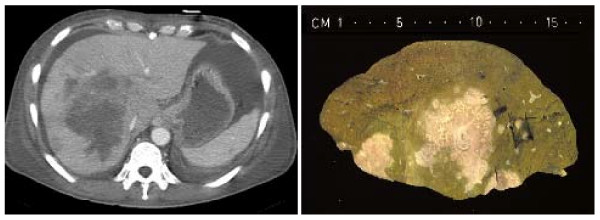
A. CT scan of the abdomen showing a large mass central in the liver, with extension to the right liver lobe. B. Postmortem aspect of the liver, with extensive fungal infection.
